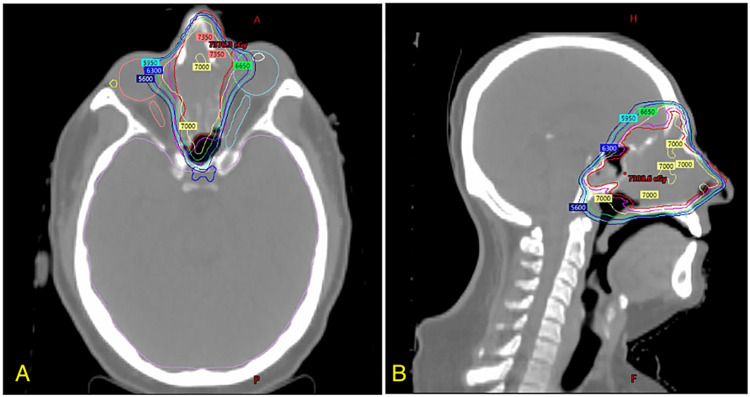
Radiation treatment plan axial (A) and sagittal (B) CT images with radiotherapy isodose lines
